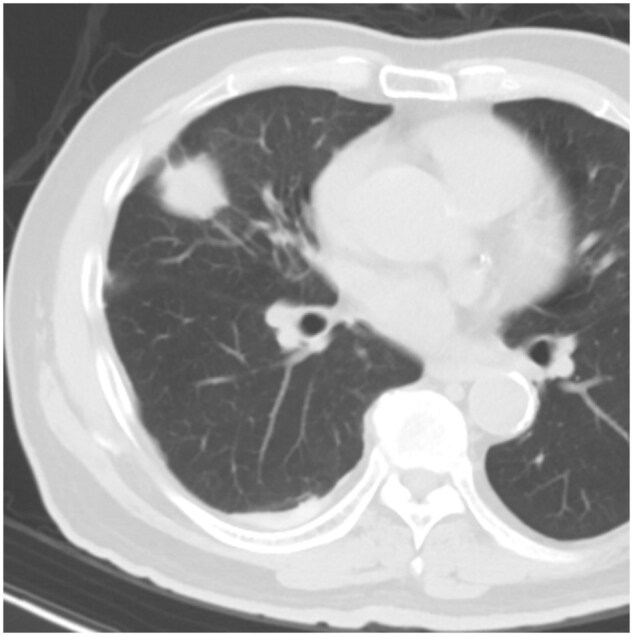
Follow-Up CT Scans Were Taken at 3 Months, Showing a Completely Healed BPF With Interval Contraction of the Ablation Zones. Abbreviation: BPF, bronchopleural fistula.
